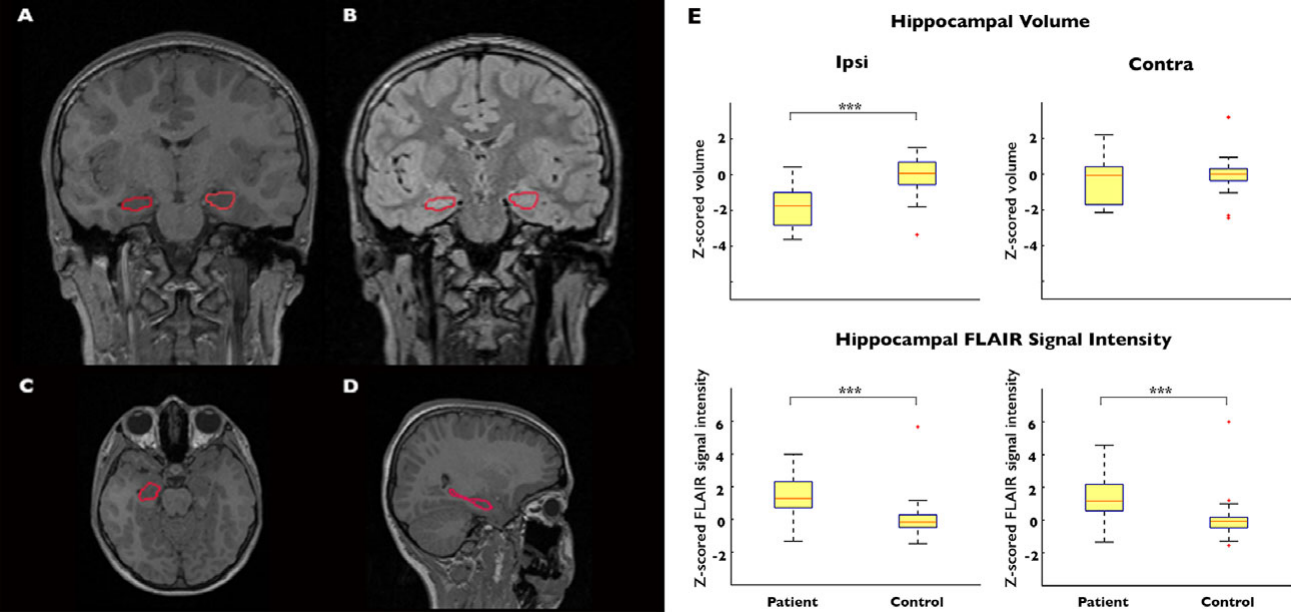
Hippocampal atrophy and FLAIR signal intensity. Hippocampal segmentations: coronal section of T1‐weighted (A) and FLAIR (B) images; and axial (C) and sagittal (D) T1‐weighted sections in a healthy control subject. E) Reduced ipsilateral but not contralateral hippocampal volume in patients. Increased normalized FLAIR signal of ipsilateral and contralateral hippocampi in patients.
